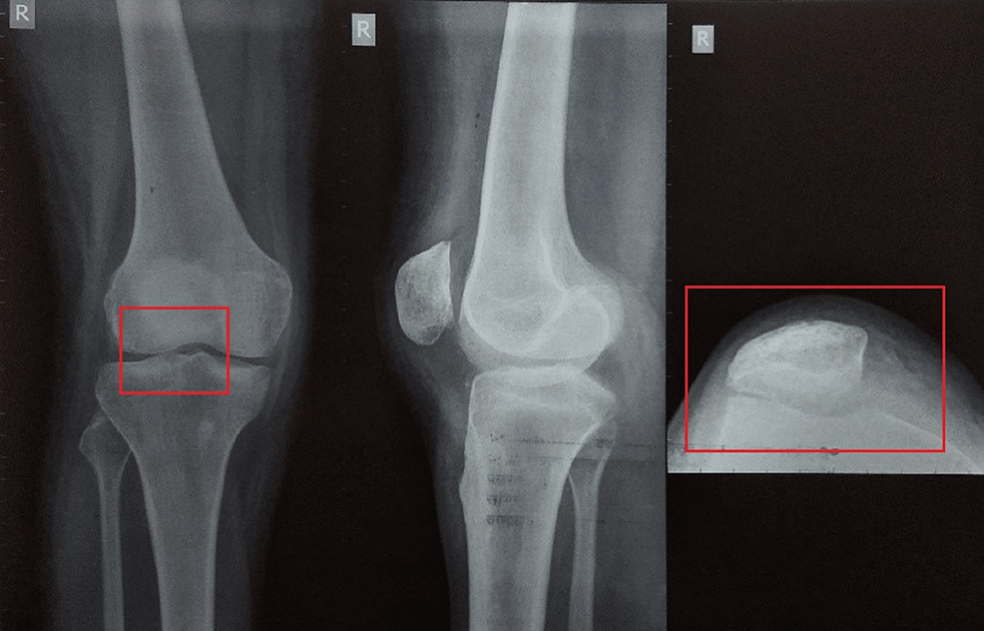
X-ray of the anterior aspect of knee shows lateral dislocation of patella.
